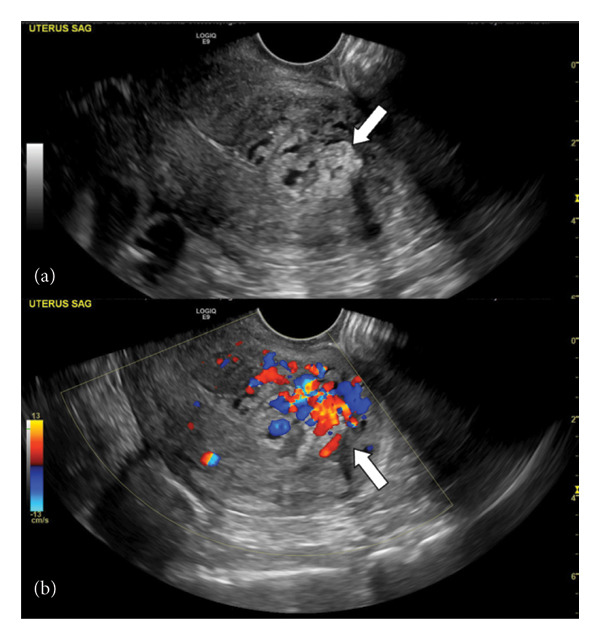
Abdominal ultrasound of the uterus (sagittal view): gray‐scale (a) demonstrates mixed echogenicity content (arrow) with color Doppler flow (b), concerning for uterine AVM.
